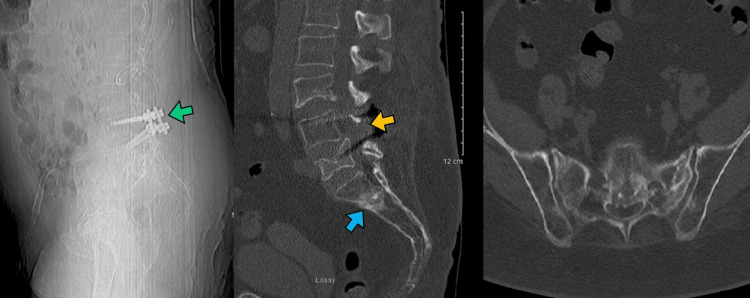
A lateral X-ray in the first image indicates a previous bilateral transpedicular fixation at the L4 and L5 levels (green arrow). The CT scan is shown in a sagittal view in the second image and in an axial view in the third image. It also reveals a prior laminectomy at L4 (orange arrow). Additionally, there are visible signs of fractures at the inferior endplate of S1 and the superior endplate of S2 (blue arrow), extending bilaterally.
